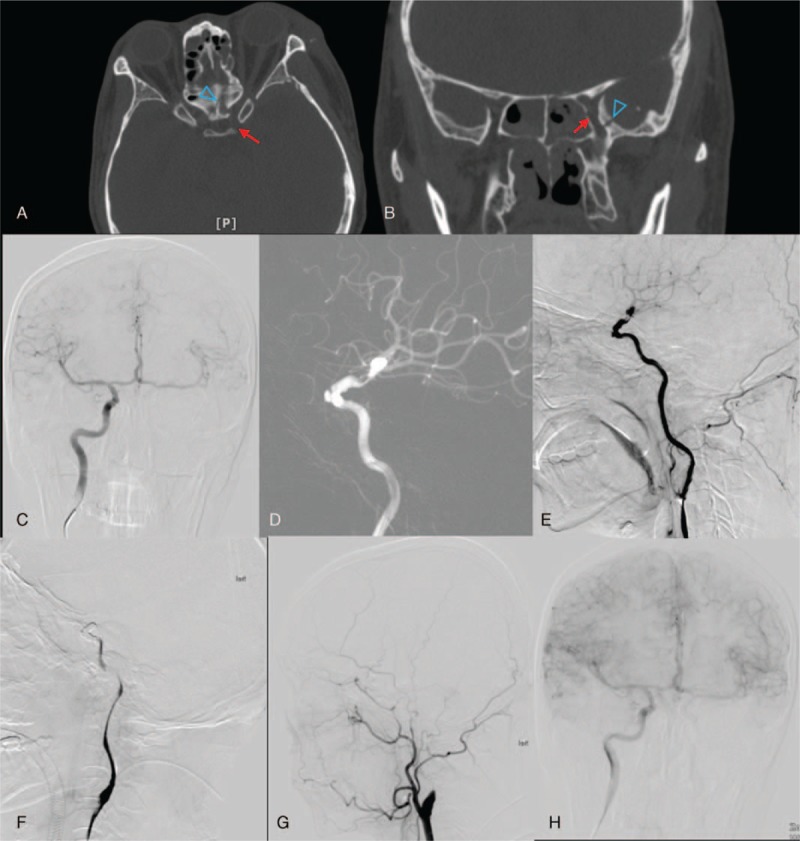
Figures from case 3. (A) and (B) The orbit computed tomography (CT) scan. The blue hollow arrow indicates fracture line and the red arrow indicates left internal carotid artery (ICA). The digital subtraction angiography (DSA) figures of right ICA (C), and left ICA (D) and (E). (F) The DSA figures after vascular occlusion. The DSA follow-up after 3 months, left ICA (G) and right ICA (H).
